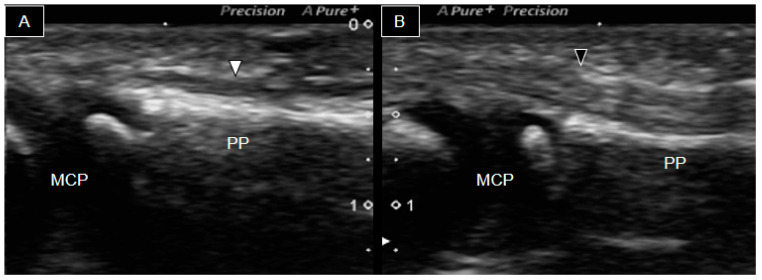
Ultrasound image of the palmar side of the fifth right (A) and left (B) fingers. The white arrowhead indicates the right finger flexor tendon, whereas the black arrowhead indicates the left flexor tendon. MCP, metacarpophalangeal joint; PP, proximal phalanx.
